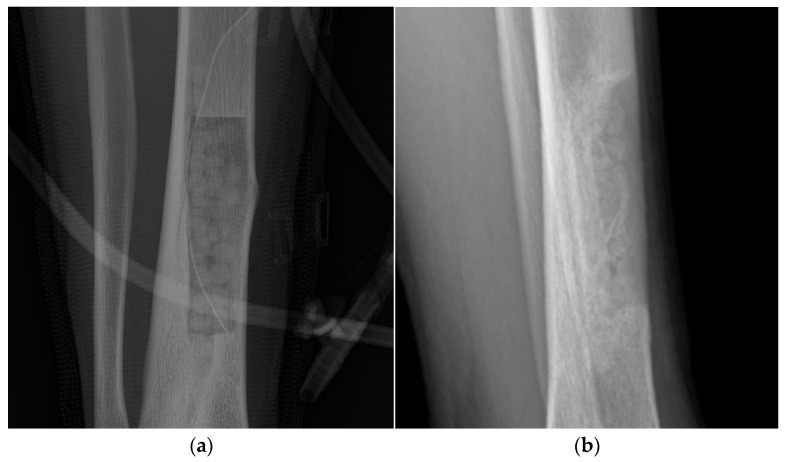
Representative radiographic images showing nHA-ATB beads in the bone cavity: (a) Immediate post-operatively; (b) 48 weeks post-operatively. The incorporation of nHA-ATB beads with surrounding bone and no translucency which might be suggestive of infection recurrence was observed.
